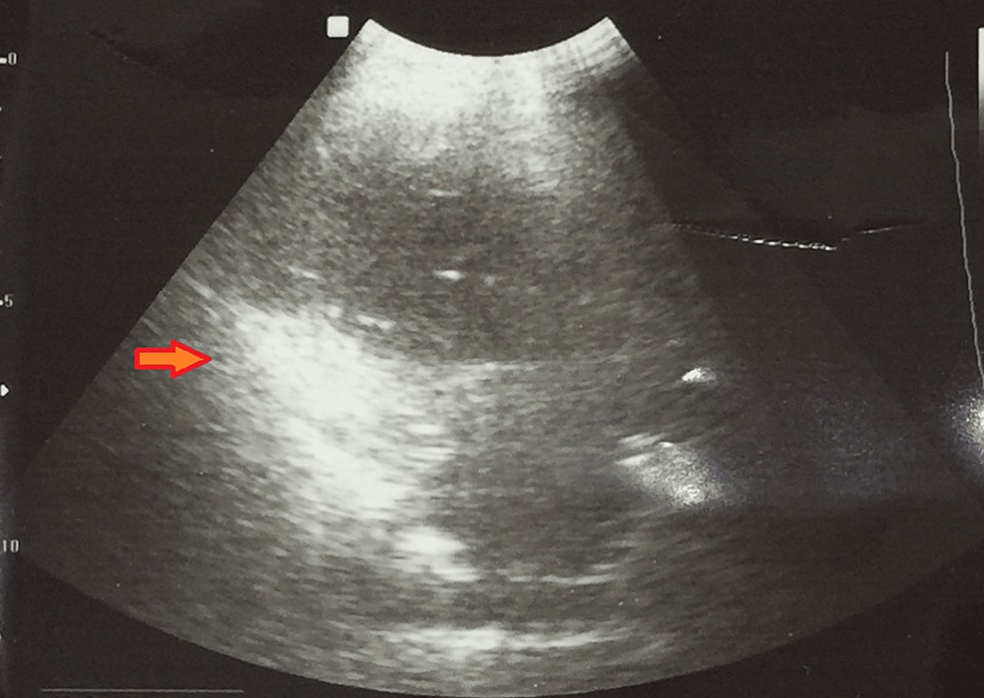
An ultrasound of the chest showing right-sided pleural effusion
